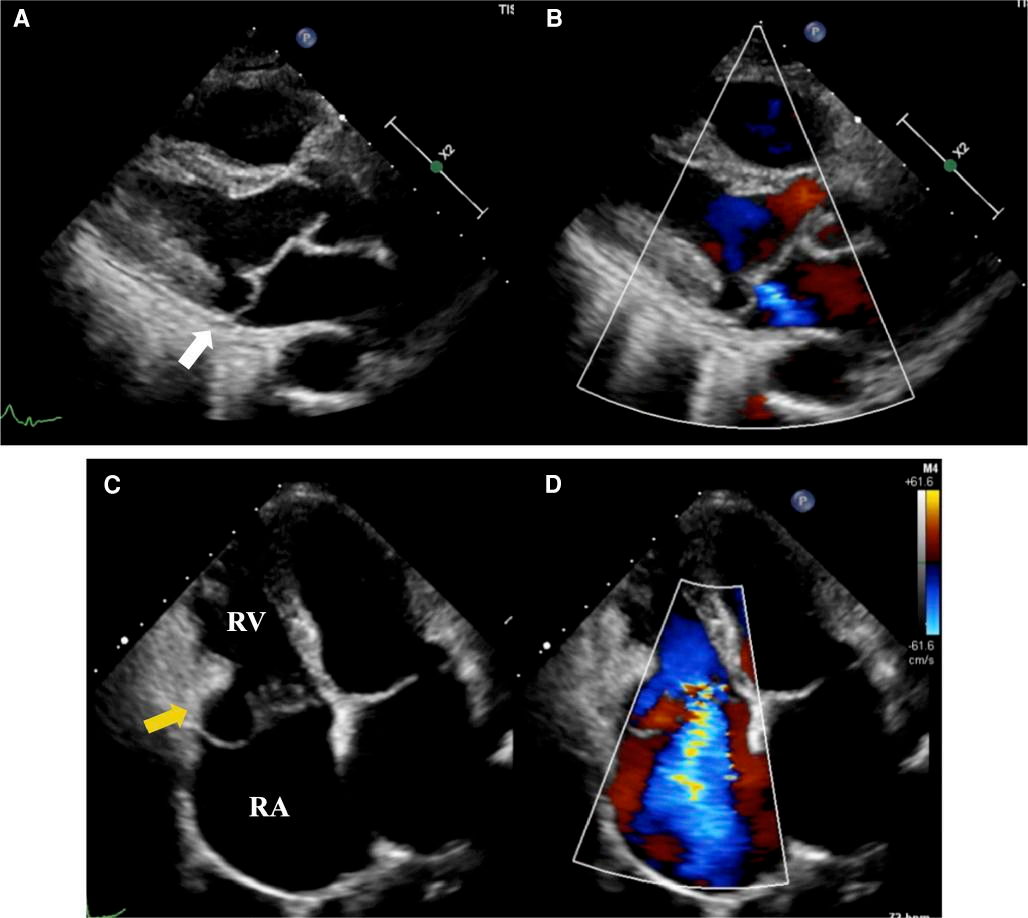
Transthoracic echocardiography images of the patient before surgery. Mitral annular disjunction in the posterolateral wall (white arrow). (*B*) Mild mitral regurgitation. (*C*) Tricuspid annular disjunction in the right ventricular free wall (yellow arrow). (*D*) Severe tricuspid regurgitation. The yellow arrows indicate areas of mitral annular disjunction. RV, right ventricle; RA, right atrium.
